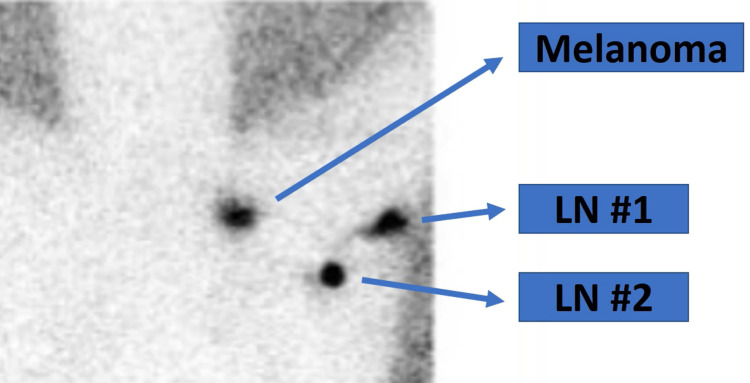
Preoperative lymphoscintigram LN: lymph node
